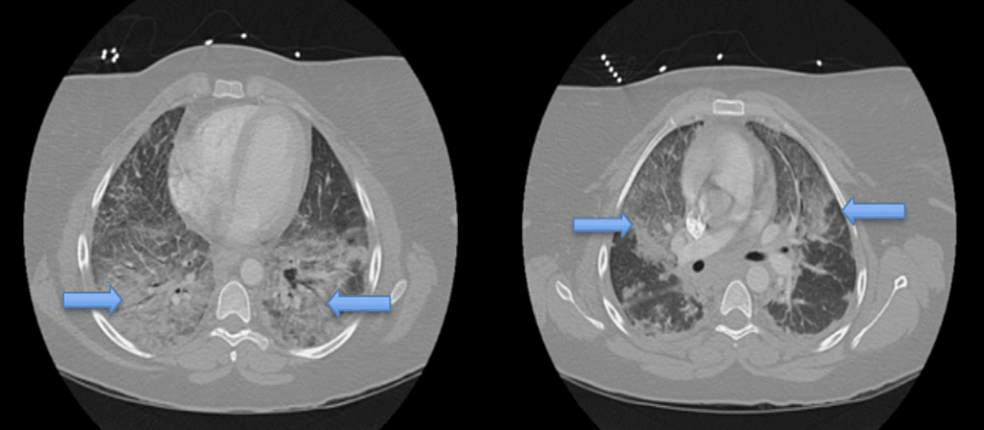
CT chest axial view CT chest axial views are remarkable for bilateral airspace opacities concerning EVALI. Blue arrows point to extensive opacities seen throughout the lung parenchyma. EVALI- E-cigarette, or vaping, product use-associated lung injury
